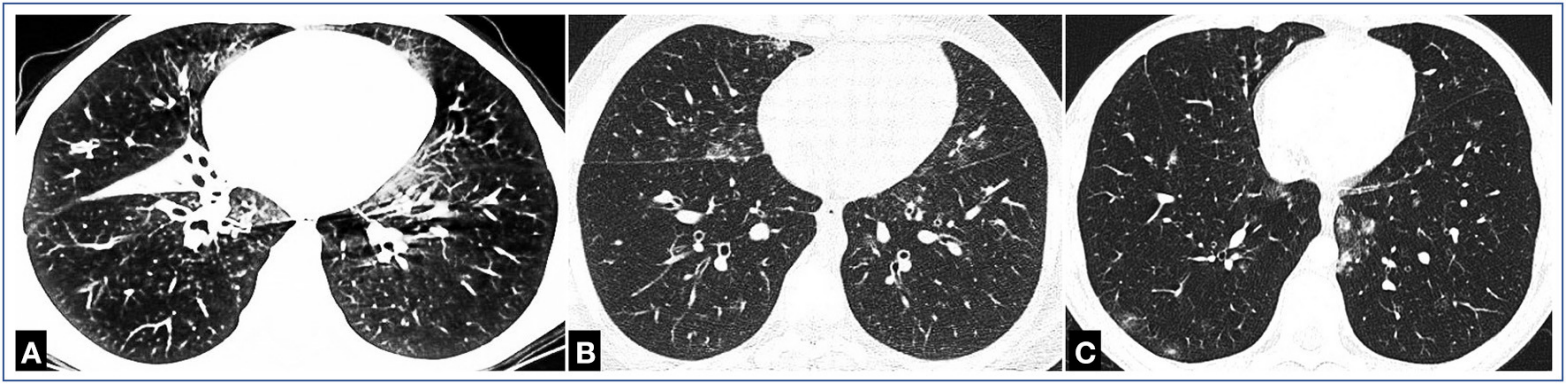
Thoracic computed tomography (CT) imaging of patients with CVID with granulomatous lymphocytic interstitial lung disease (GLILD). (A) Multiple confluent nodules, tree-in-bud pattern reflecting bronchiolitis, interstitial pneumonia, peribronchial thickening and bronchiectasis, atelectasis, and fibrosis of the right middle lobe. (B) Patchy, confluent ground-glass opacities, tree-in-bud pattern, single nodules. (C) Multiple, irregular, disseminated perivascular and subpleural nodules, ground-glass opacities.
